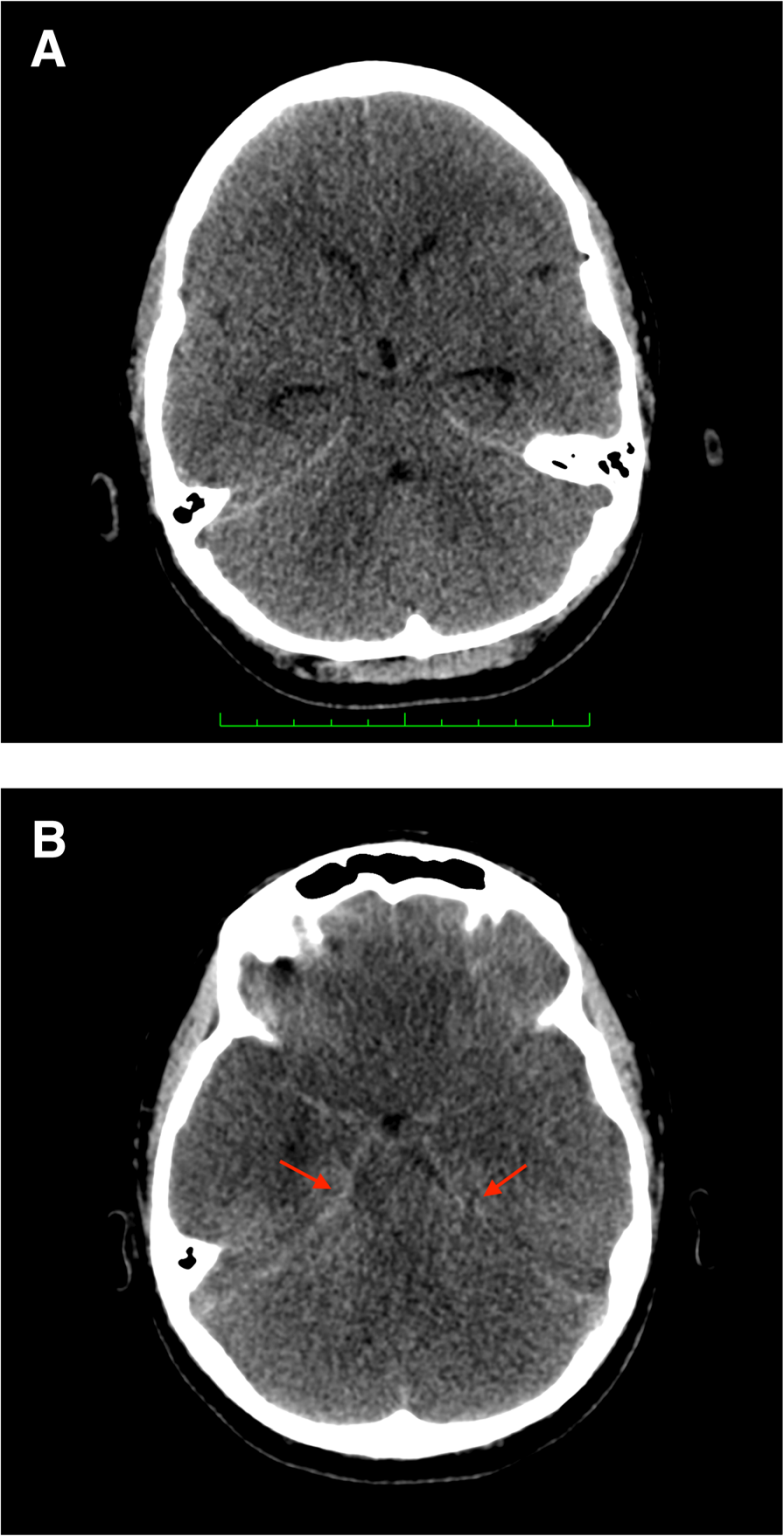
a Non-contrasted cranial CT, on day of admission, taken at the level of the basal cisterns, in axial section showing evidence of cerebral edema, with mild loss of grey-white matter differentiation. b Non-contrasted cranial CT, on day 3 of admission, taken at the level of the basal cisterns, showing evidence of high density areas (red arrows) indicative of pseudo-SAH. Note that the cerebral edema is worse compared to the initial CT (a)
